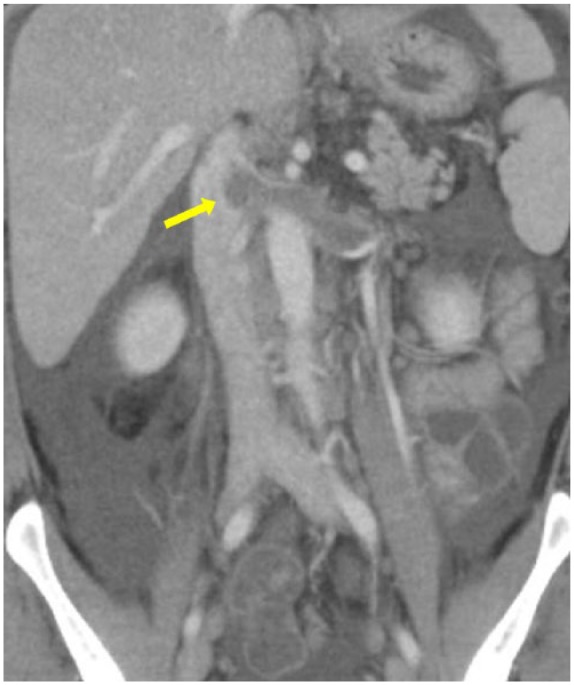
Computed tomography scan of abdomen/pelvis with contrast. Left renal vein thrombus with total luminal occlusion and extension into the inferior vena cava (yellow arrow), along with diffuse lymphadenopathy (periportal, mesenteric, retroperitoneal), hepatomegaly, and splenomegaly.
